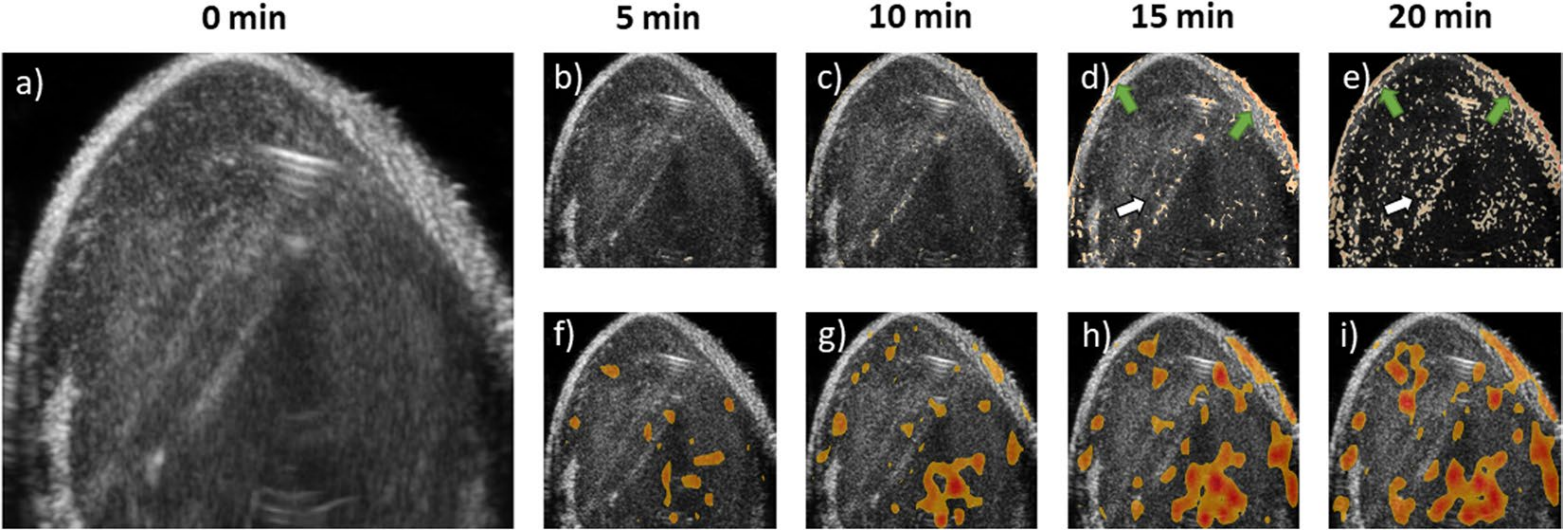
(a) Liposomal contrast in tumors at time 0; (b–e) Spatial ultrasound mapping after injection (5–20 min) based on conventional methods, with pixel by pixel subtraction for the motion compensated ultrasound images showed high noise; (f–i) On-the-fly maps of liposomal contrast/accumulation generated by SFF-US imaging technique (5–20 min) resist the effects of noise to pinpoint liposome accumulation.
